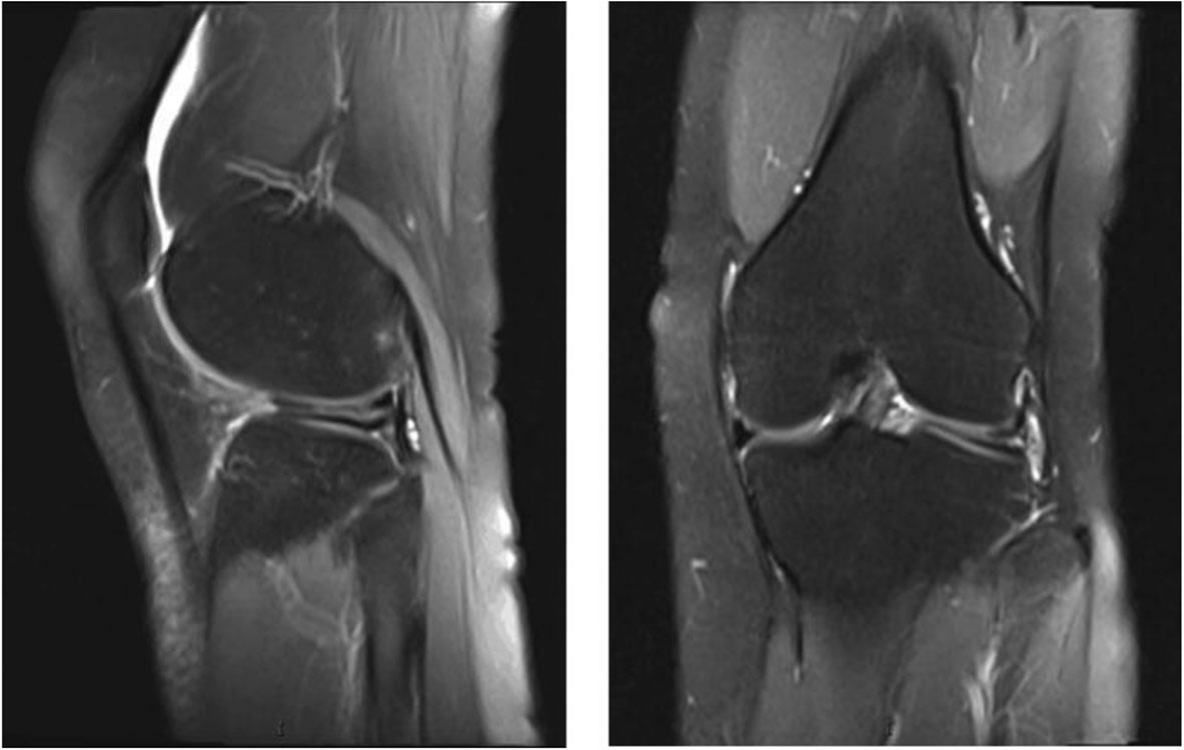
MRI demonstrates a horizontal discoid lateral meniscus tear
